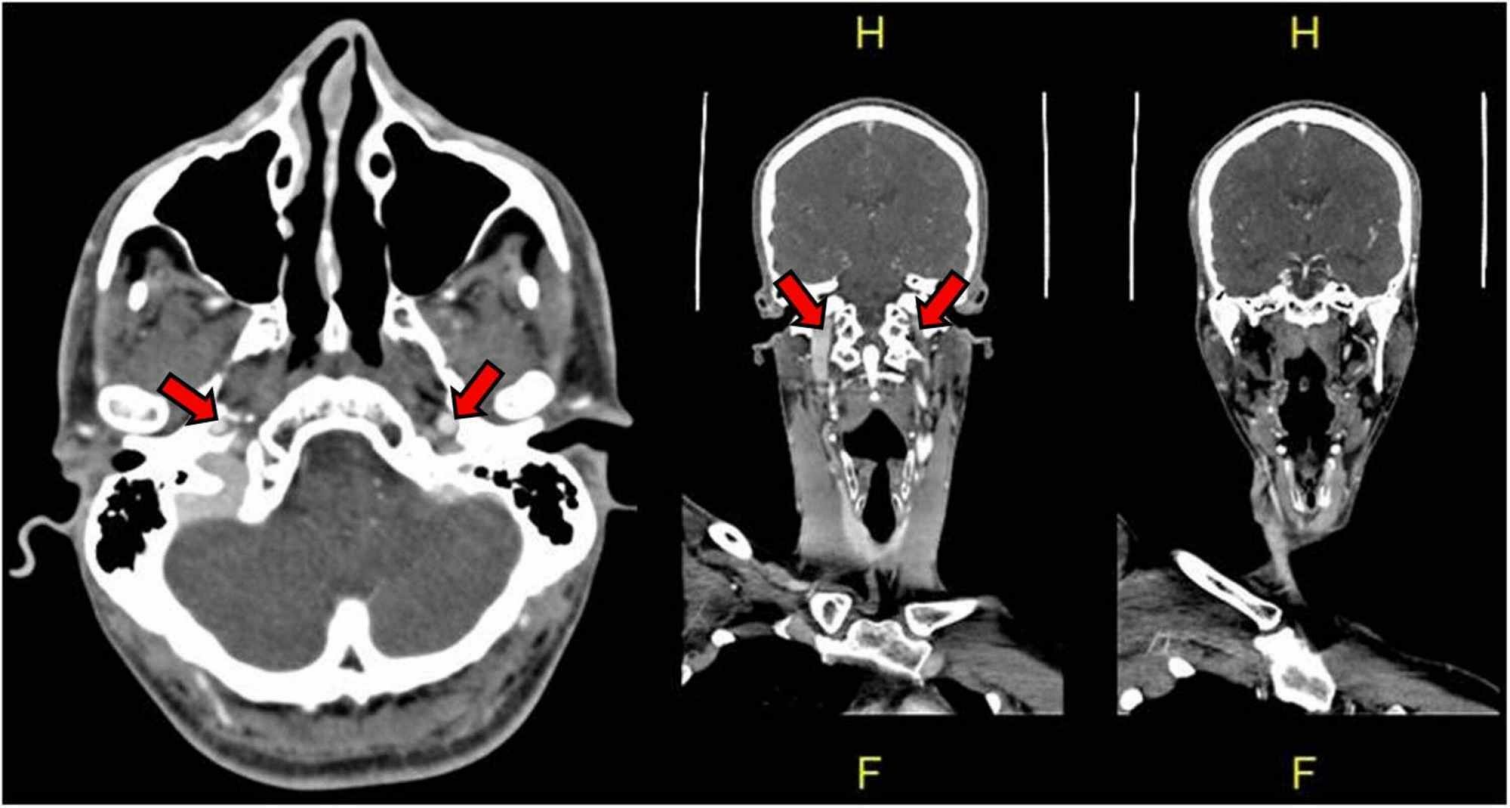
Computed tomography angiogram (CTA) of the vertebral arteries CTA reveals that the distal bilateral vertebral arteries (VA) are thrombosed to the middle of the basilar artery (BA), sparing the left anterior inferior cerebellar artery (AICA) and other parts of the circle of Willis including both posterior communicating arteries.
